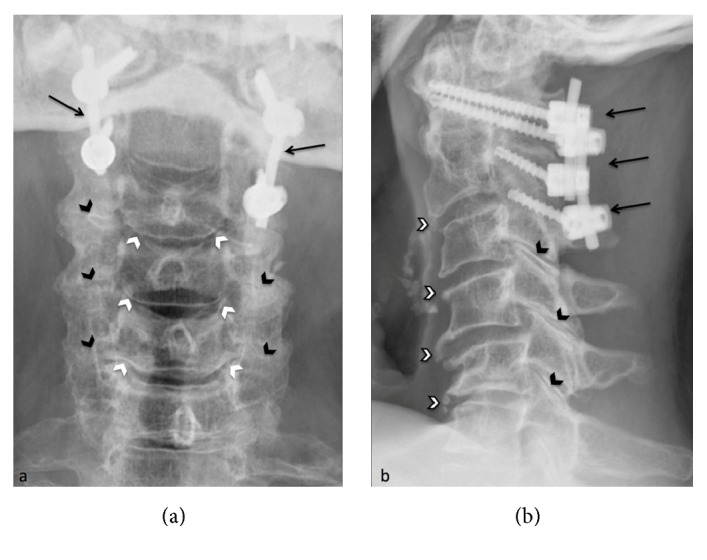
Postoperative X-rays of the cervical spine are also acquired for the follow-up. We see the posterior C1–C2 fixation with transarticular screws (a,b, black arrows). These X-rays show better degenerative changes of the cervical spine; disco-uncarthrosis (a, white arrowheads), interarticular posterior staggered arthrosis (a, b, black arrowheads), and anterior marginal osteophytosis (b, black-framed white arrowheads).
